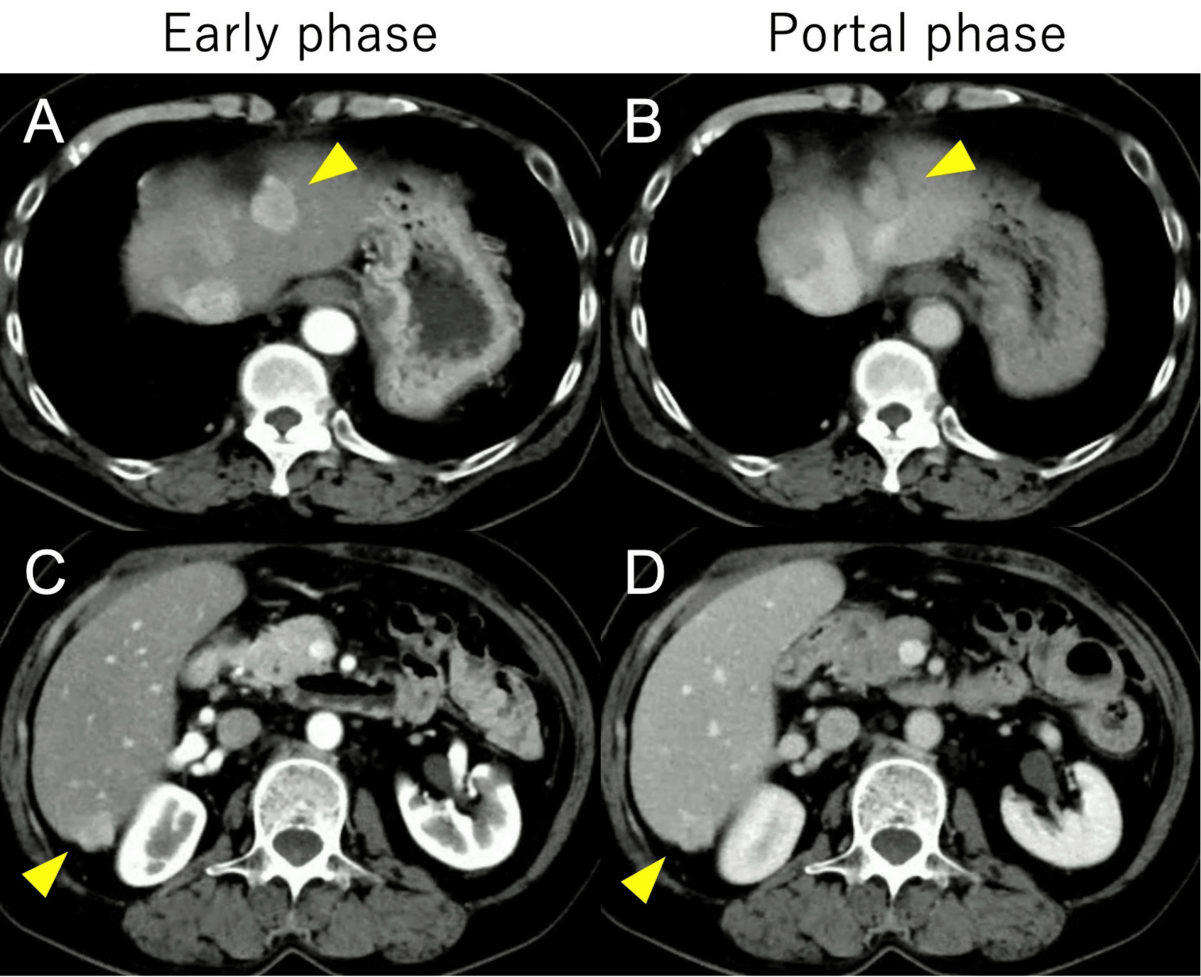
Dynamic CT images of the liver (A, B) Early arterial phase (A) and portal phase (B) of the upper abdomen, show a homogeneously enhancing mass in segment 2 of the liver (yellow arrowheads). The lesion demonstrates higher attenuation compared to the surrounding liver parenchyma in the early phase (A) and becomes relatively hypoattenuating in the portal phase (B). (C, D) Early arterial phase (C) and portal phase (D) of the lower abdomen, reveal a similar enhancing mass in segment 6 of the liver (yellow arrowheads). This lesion also shows hyperattenuation in the early phase (C) and relative hypoattenuation in the portal phase (D). Both lesions demonstrate enhancement patterns mimicking the washout typically seen in hepatocellular carcinoma, with hyperenhancement in the early arterial phase and relative hypoattenuation in the portal venous phase compared to the surrounding liver parenchyma.
